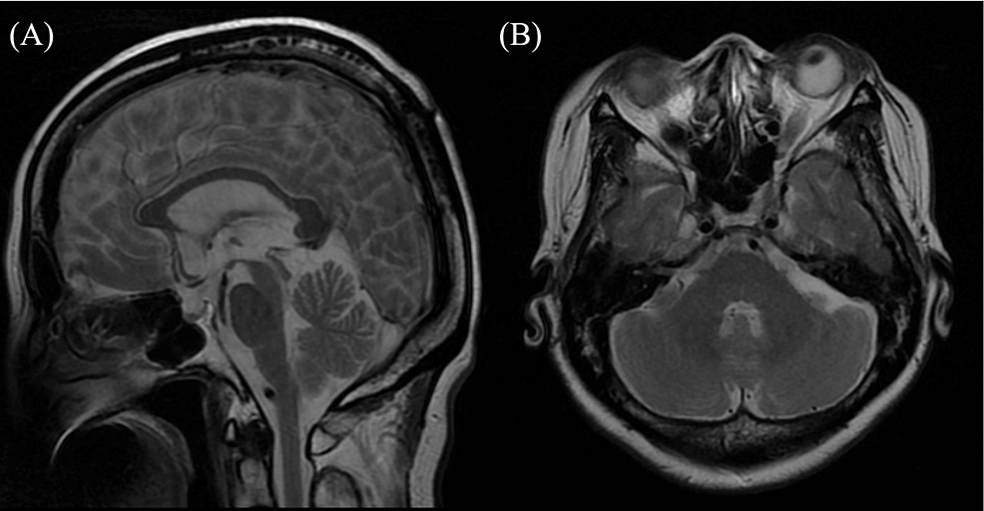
The result of brain magnetic resonance imaging (MRI) (A, B) T2-weighted brain MRI showing that atrophy was unremarkable in the brainstem or cerebellum (A) sagittal section; (B) axial section
